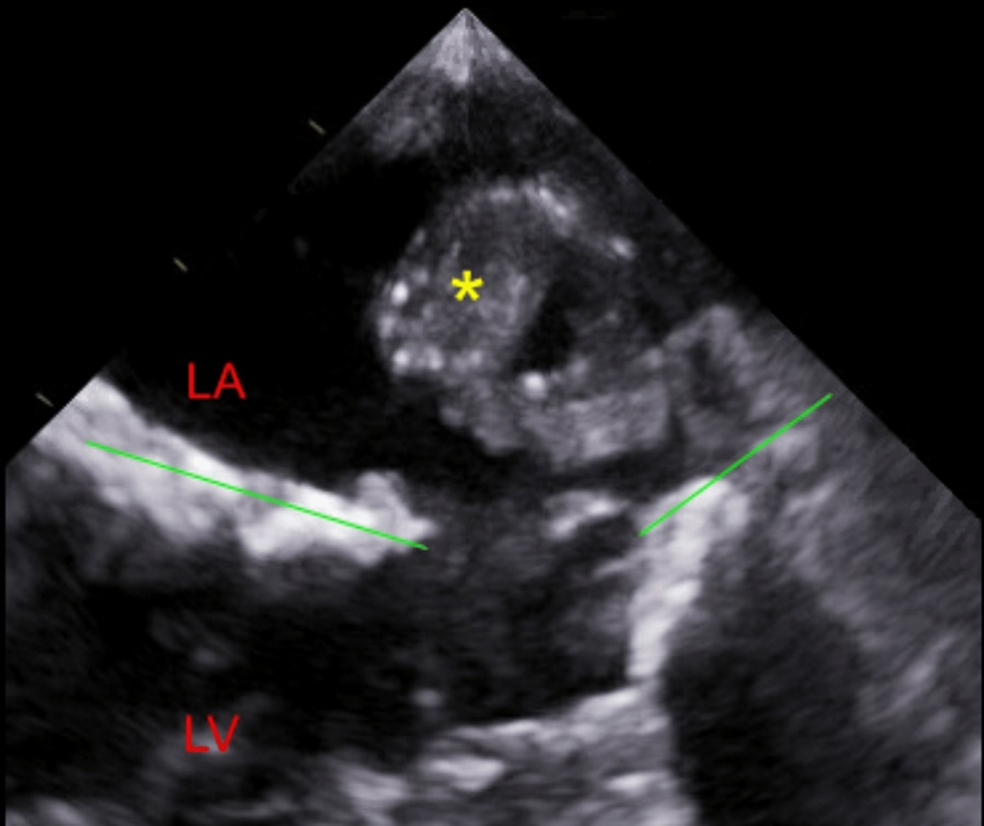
Image from transesophageal echocardiogram showing a large vegetation (yellow asterisk) attached to the posterior leaflet of the mitral valve.
